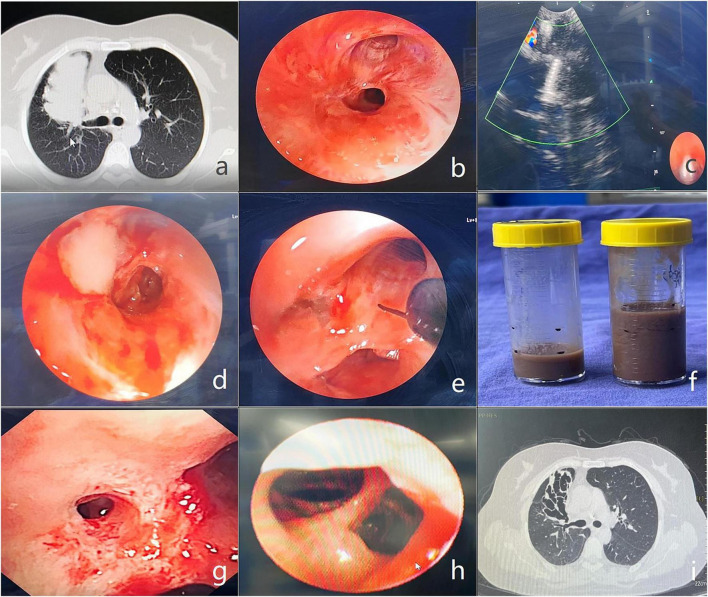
(a) Chest CT revealed consolidation in the right upper lobe; (b) bronchoscopy revealed complete occlusion of the right upper lobe anterior segment bronchus; (c) endobronchial ultrasound-guided transbronchial needle aspiration (EBUS-TBNA) was performed with meticulous avoidance of perilesional blood vessels; (d) purulent secretion was noted oozing from the puncture site; (e) the overlying scar tissue at the puncture site was incised using an electrical knife; (f) a large volume of brown, viscous secretion was drained; (g) the bronchial incision was further enlarged; (h) distal bronchiectasis was noted via an ultra-thin bronchoscope; (i) follow-up chest CT revealed the disappearance of consolidation in the right upper lobe.
